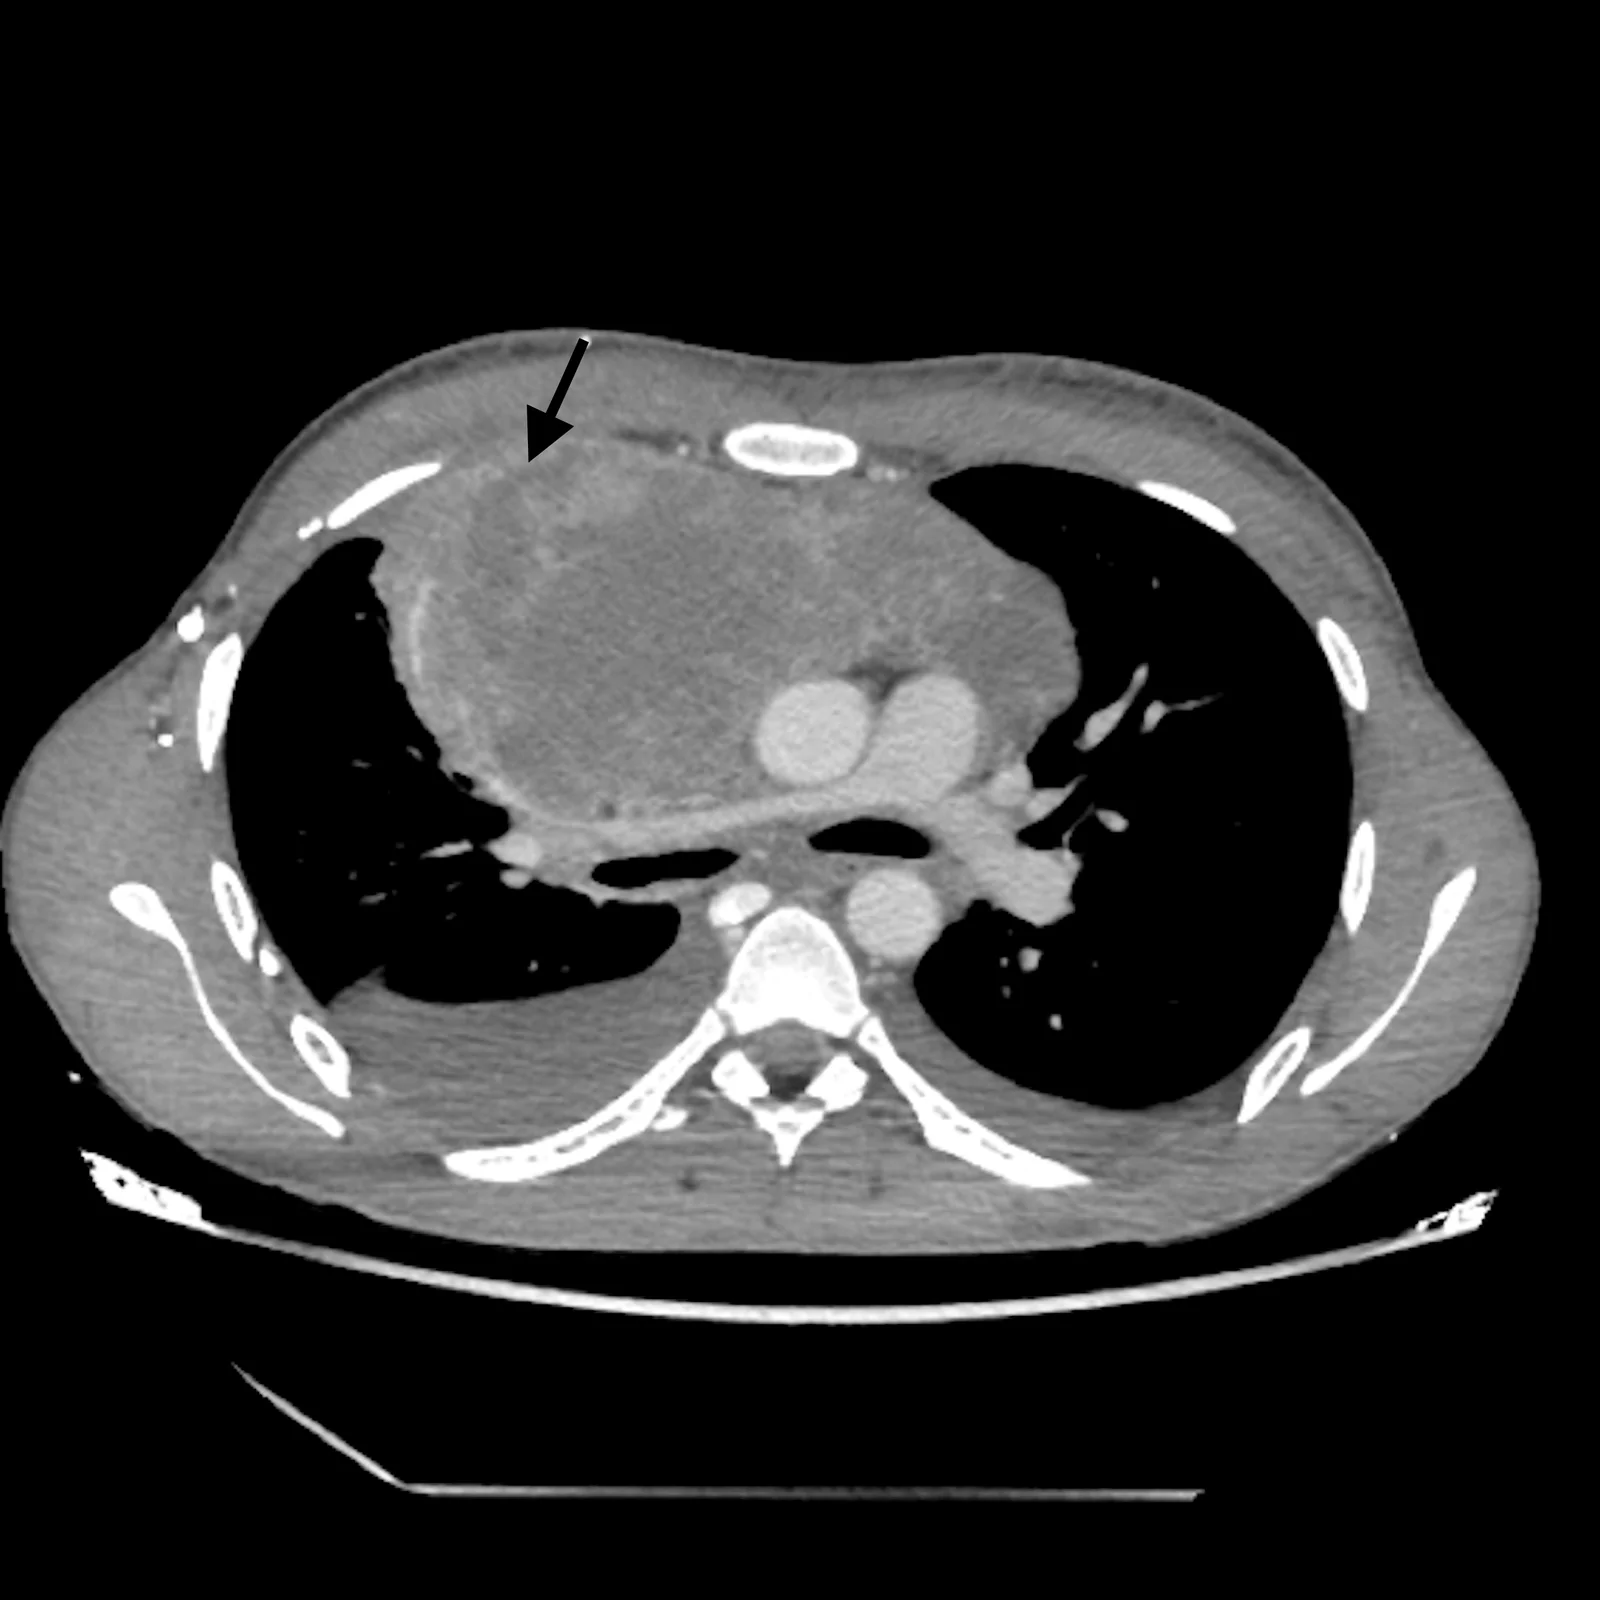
Contrast-enhanced CT chest showing anterior mediastinal mass encasing SVC (arrow) CT: computed tomography; SVC: superior vena cava
